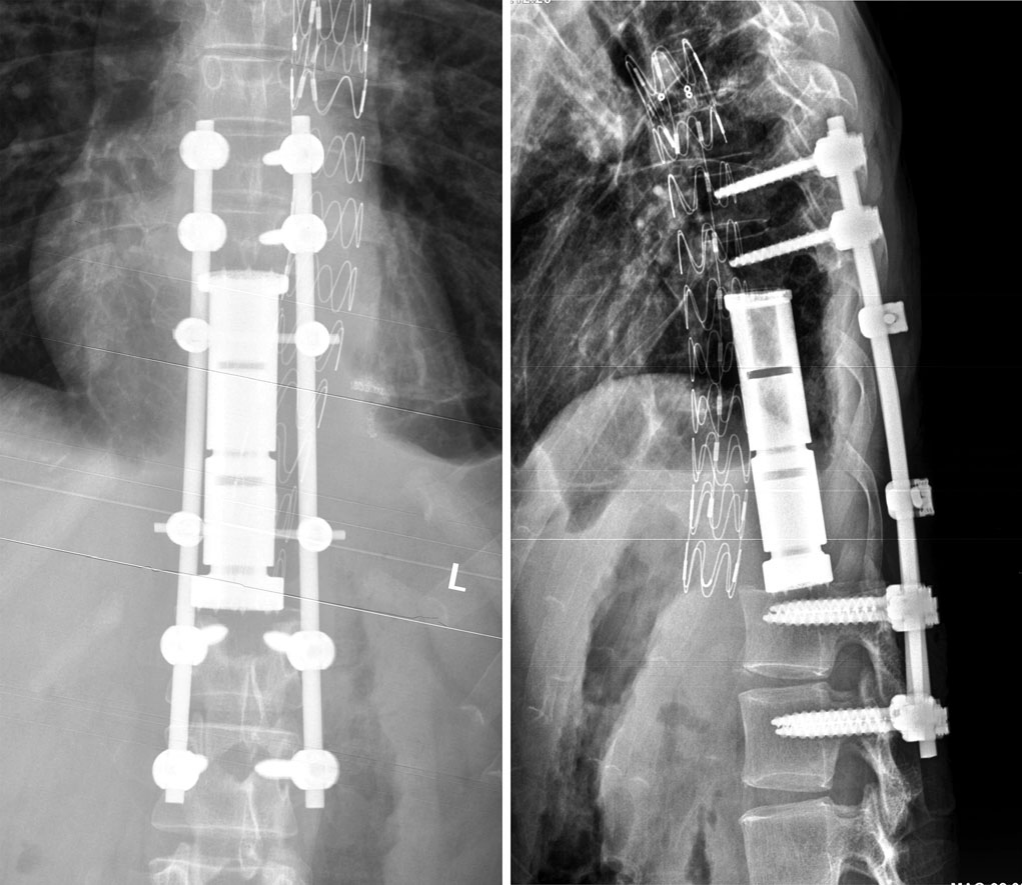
A patient (No. 16) with spinal GCT underwent TES and reconstruction with the 3D‐printed modular vertebral prosthesis. Postoperative plain radiograph.
